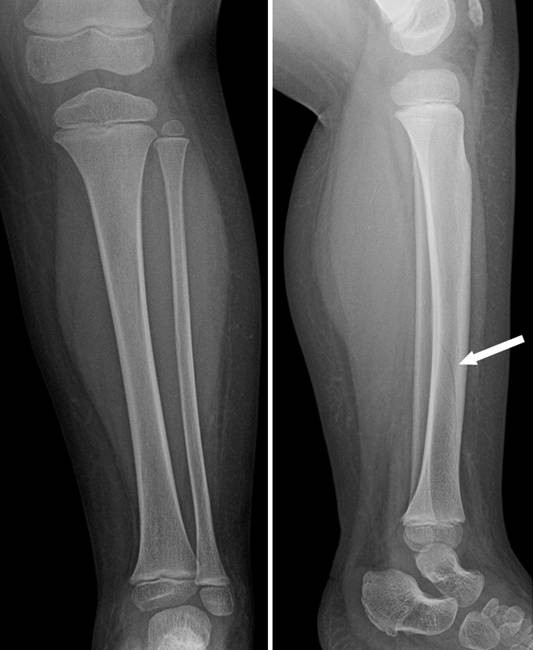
Whole-leg radiograph of the lower extremity revealed a subtle non-displaced oblique tibia fracture which was only seen on the lateral view (*arrow*).
